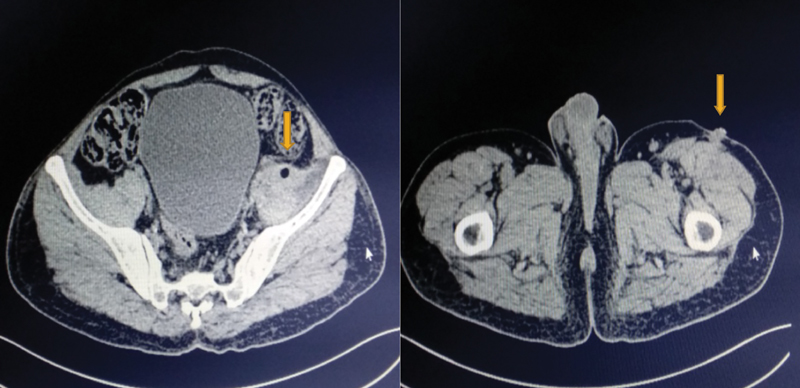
Contrast-enhanced computed tomography showing left-sided colonic fistula communicating with a cutaneous opening in thigh.
